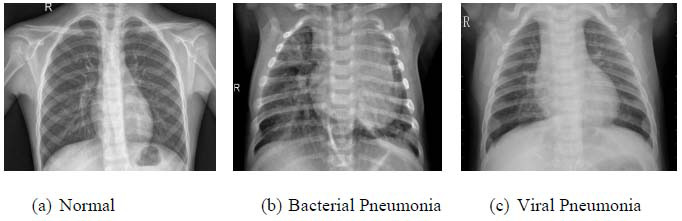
Chest X-ray images of pneumonia patients.
